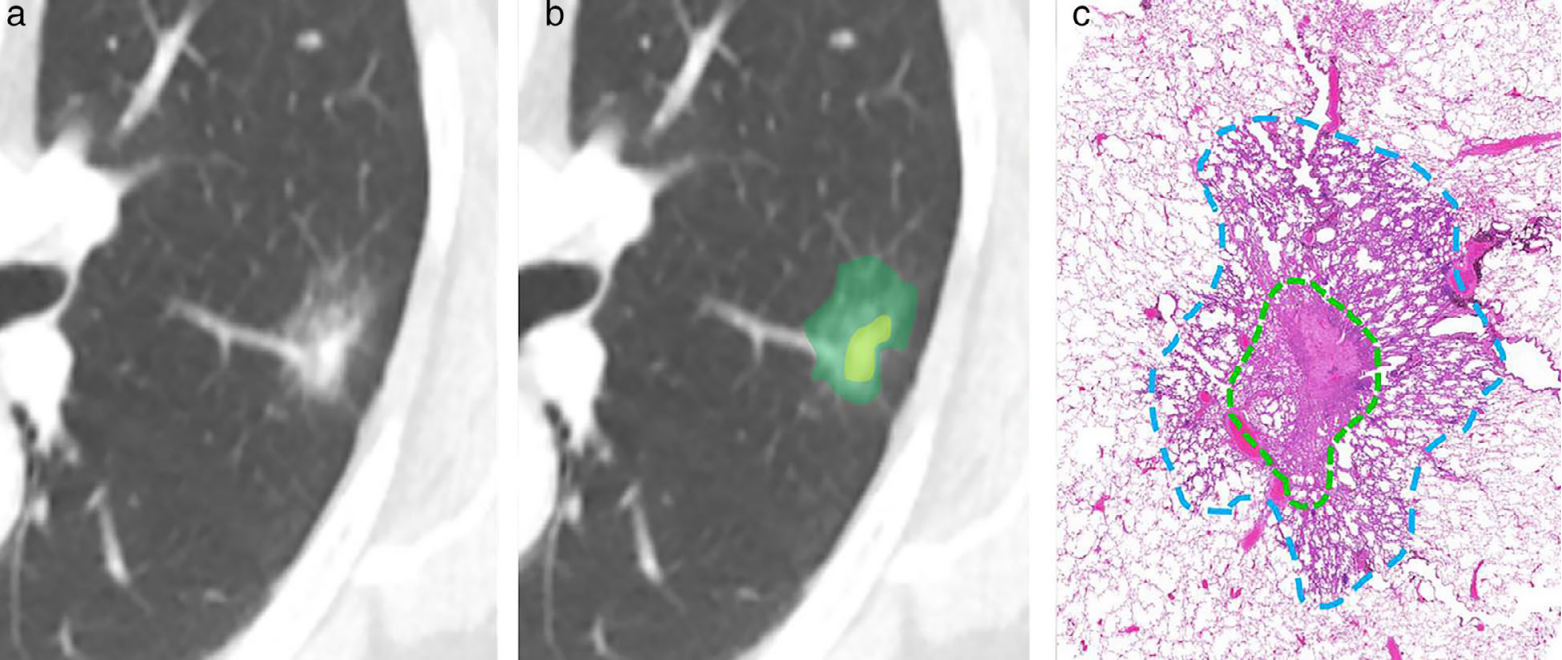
(a and b), representative computed tomography images showing measurement of whole tumor size (green) and sold tumor size (yellow) of part‐solid nodule. (c), Blue dotted line shows whole pathologic size, and green dotted line represents invasive tumor component.
